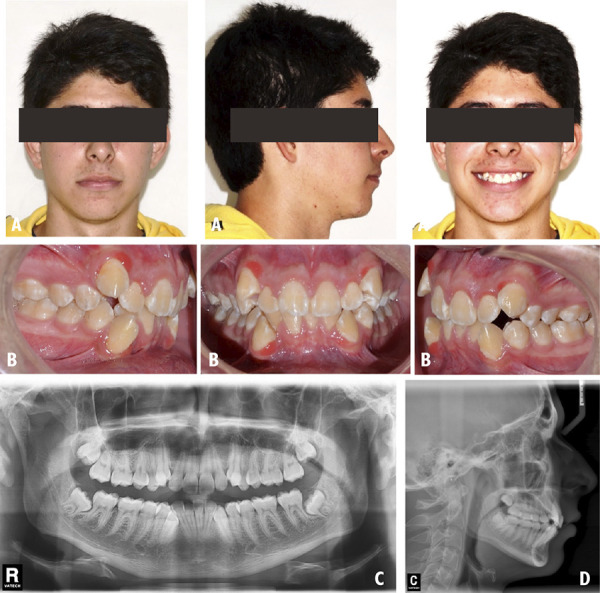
Case 2. Initial pre-treatment records. A: Extraoral photographs. B: Intraoral photographs. C: Panoramic radiograph. D: Cephalometric radiograph.
